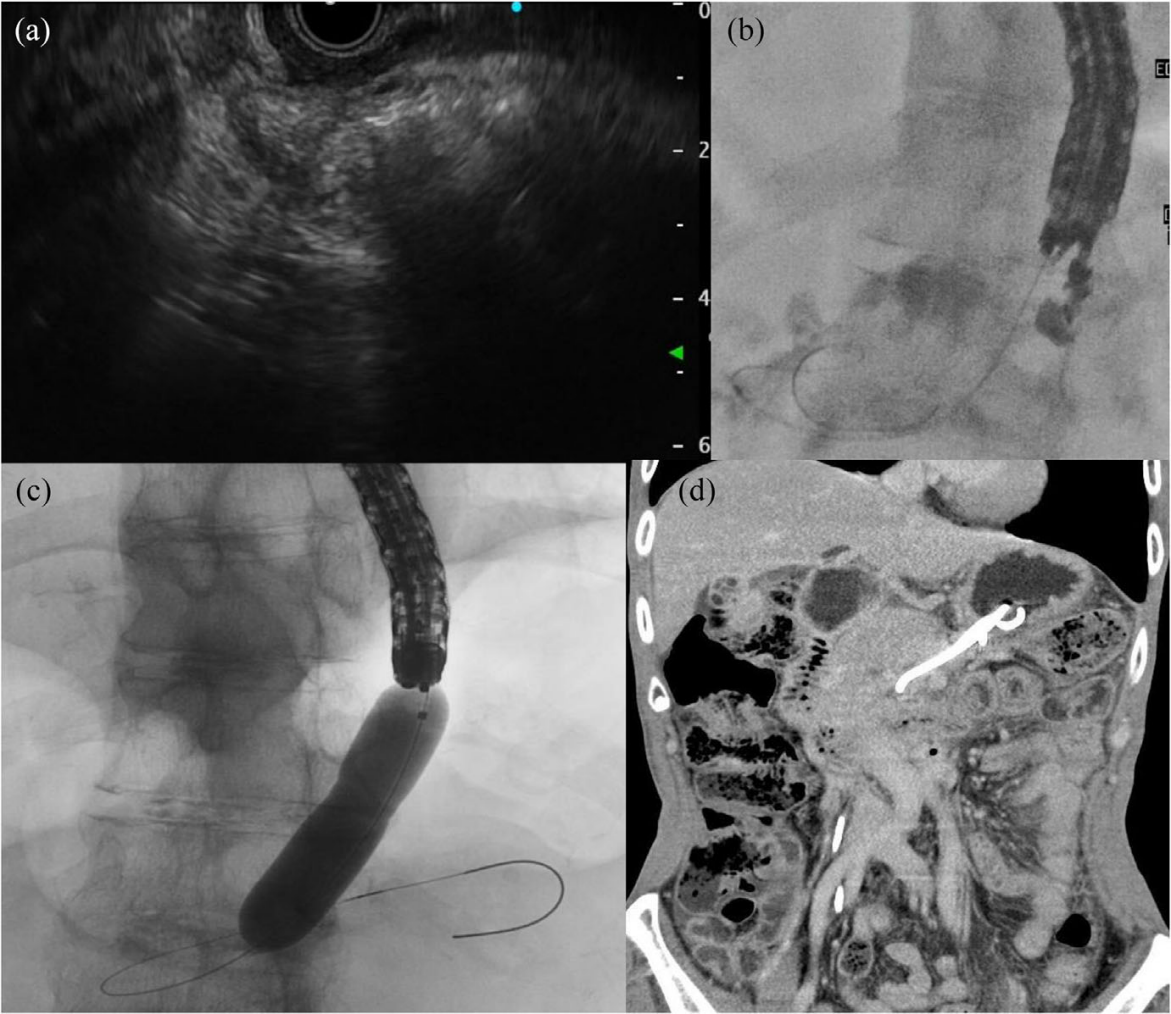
(a and b) Repeat EUS‐CD confirmed that the ENBD tube was successfully placed in the WON. EUS‐CD, endoscopic ultrasound‐guided cyst drainage; ENBD, endoscopic nasobiliary drainage; WON, walled‐off pancreatic necrosis. (c) Necrosectomy was performed by dilating the fistula with a biliary dilatation balloon. (d) This is after the necrosectomy is completed.
